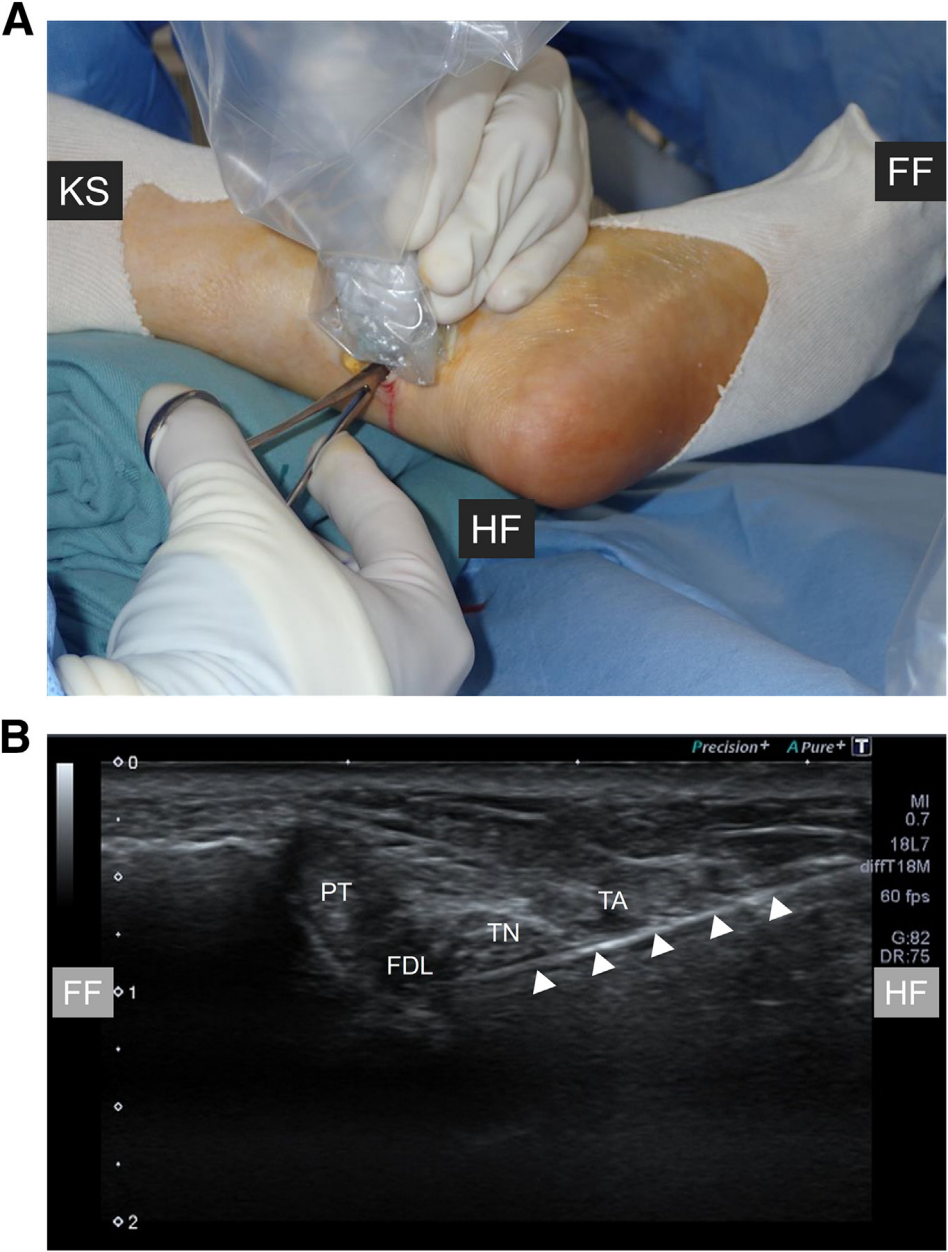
Blunt dissection of the left foot using mosquito Pean forceps under ultrasonographic guidance. (A) A mosquito Pean forceps is inserted from a medial approach while the patient remains supine. (B) The instrument (arrowheads) is shown clearly separating the neurovascular bundle and flexor hallucis longus tendon under ultrasound. (FDL, flexor digitorum longus tendon; FF, forefoot side; HF, hindfoot side; KS, knee side; PT, posterior tibial tendon; TA, tibial artery; TN, tibial nerve.)
